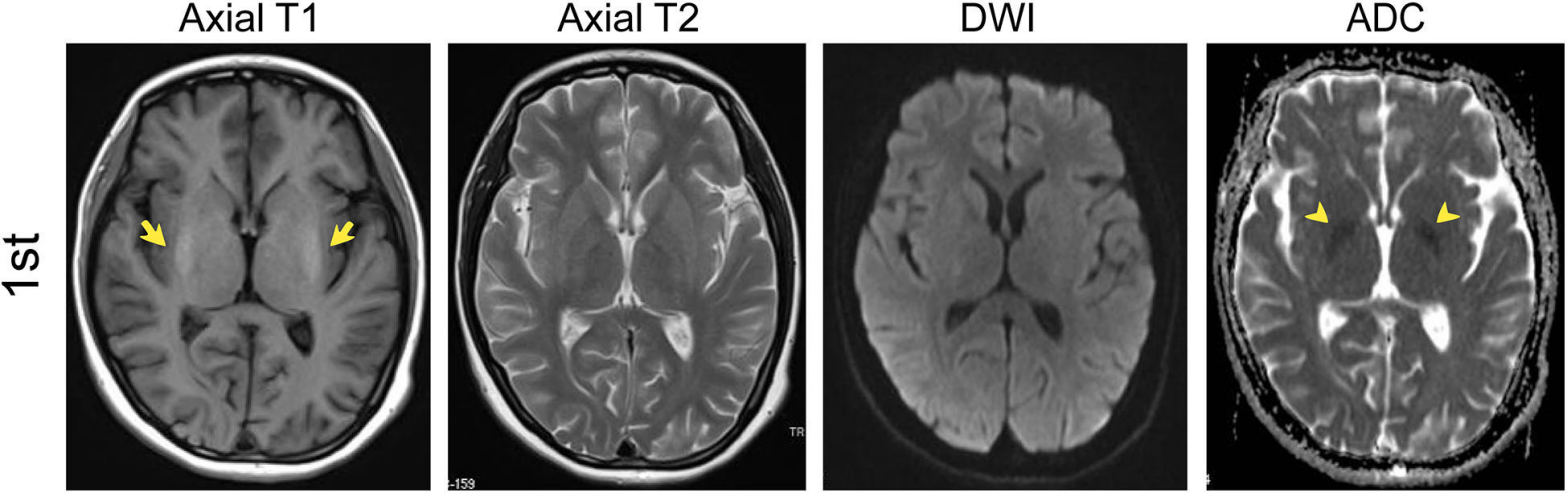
MRI results of the first admission (in September). T1, T2, DWI, and Apparent diffusion coefficient (ADC) axial images in September (1st) showed symmetric lesions in the bilateral basal ganglion with hyper-intensity (yellow arrows) and symmetric hypo-intensity of ADC in the internal capsule (yellow arrowheads).
